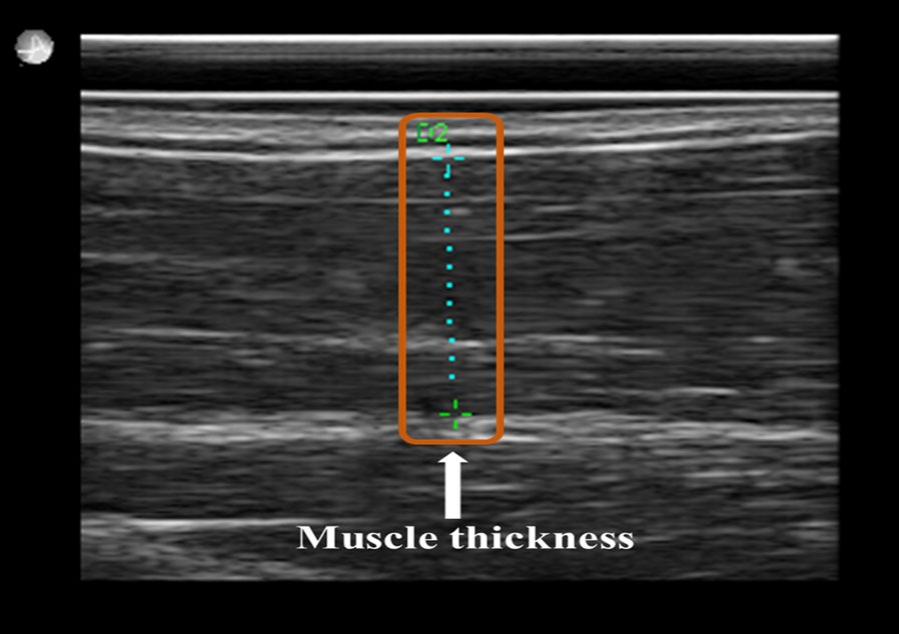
Ultrasonography measurement of bicep brachii muscle thickness at the midpoint
